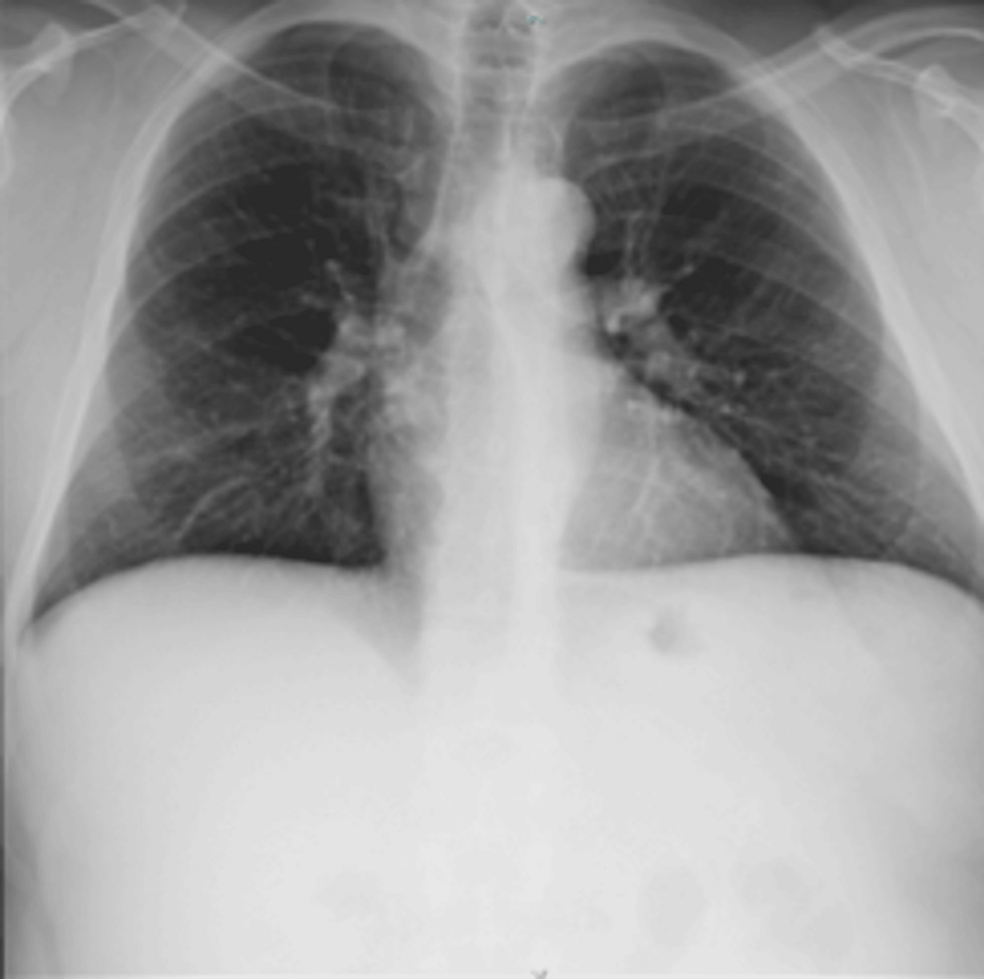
Thoracic X-ray Thoracic X-ray showing normal cardiac position.
